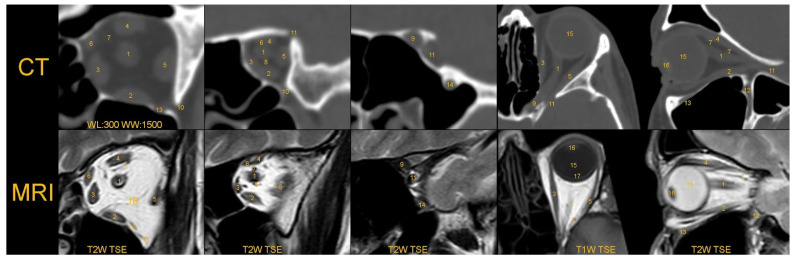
Important anatomical structures observed in the eye socket in two principal imaging methods. 1: nervus opticus, musculi recti orbitae: 2: inferior, 3-medialis, 4: superior, 5: lateralis, 6: musculus obliquus superior, 7: vena ophthalmica superior, 8: arteria ophthalmica, 9: canalis opticus, 10: fissura orbitalis inferior, 11: fissura orbitalis superior, 12: fossa pterygopatina, 13: canalis infraorbitalis, 14: foramen rotundum, 15: corpus vitreum oculi, 16: lenticula oculi, 17: retina, 18: systema ligamentaris corporis adiposi orbitae. Note the complexity of the fine structure of the ligamentous system in the fat body of the orbit revealed by MRI and the ability to observe the course of the retina in a T1-weighted sequence. Abbreviations: CT: computed tomography, MRI: magnetic resonance imaging, WL: window level in Housfield units, WW: window width in Housfield units, T2W_TSE: image of T2-weighted turbo spin echo, T1W: weighted image.
